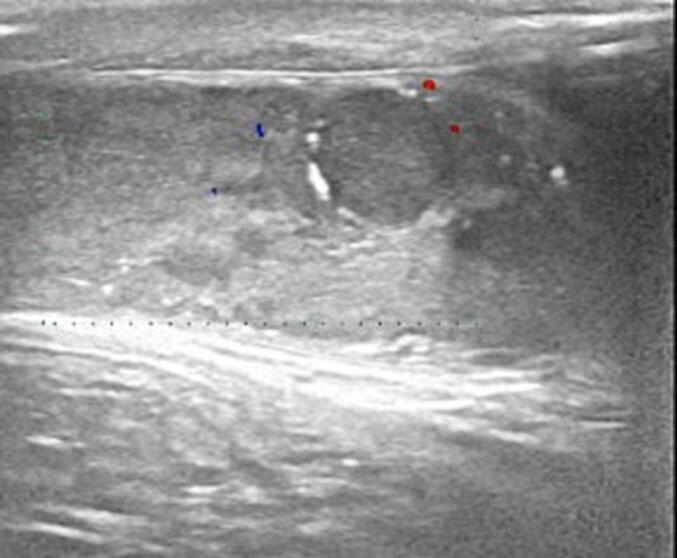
Ultrasound showed heterogenous zones on the right testicle measuring 16 mm.
